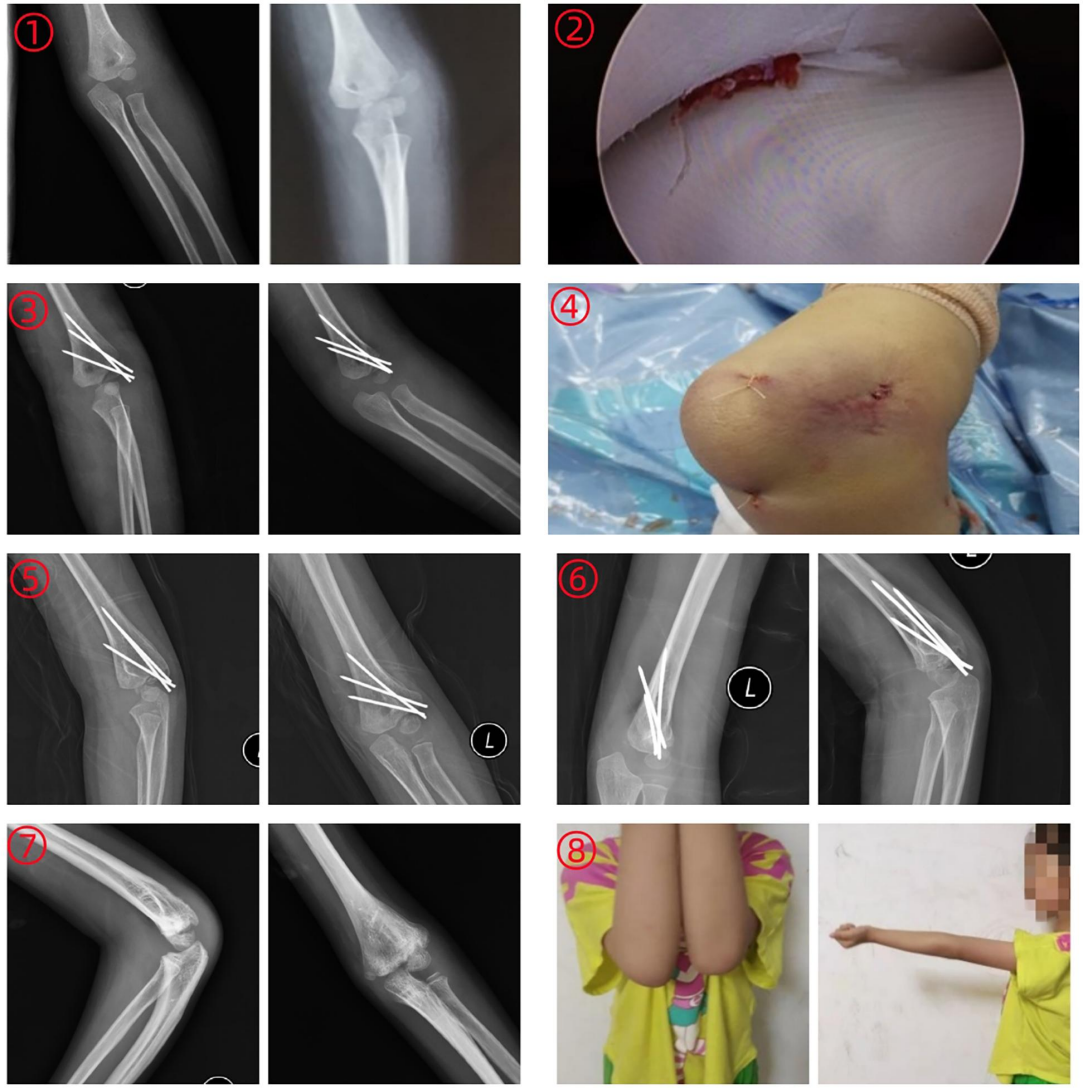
Female patient, 5 years old. (1): Preoperative anteroposterior and lateral radiographs of the left elbow joint showing lateral condylar fracture of humerus with posterolateral displacement of the distal fragment; (2): Intraoperative imaging following arthroscopic reduction of the lateral humeral condyle; (3): Postoperative review confirming anatomical reduction and proper fixation of the lateral humeral condyle fragment; (4): Postoperative incision appearance. (5): One-month postoperative x-ray review revealed satisfactory fracture reduction with callus formation at the fracture site. (6): Three-month postoperative x-ray review demonstrated continuous callus bridging the fracture line with obliteration of the fracture line, indicating fracture healing. (7): Two-year postoperative x-ray review confirmed bony union of the lateral condylar fracture of humerus without significant varus or valgus deformity. (8): Elbow joint functional assessment at 2-year follow-up.
